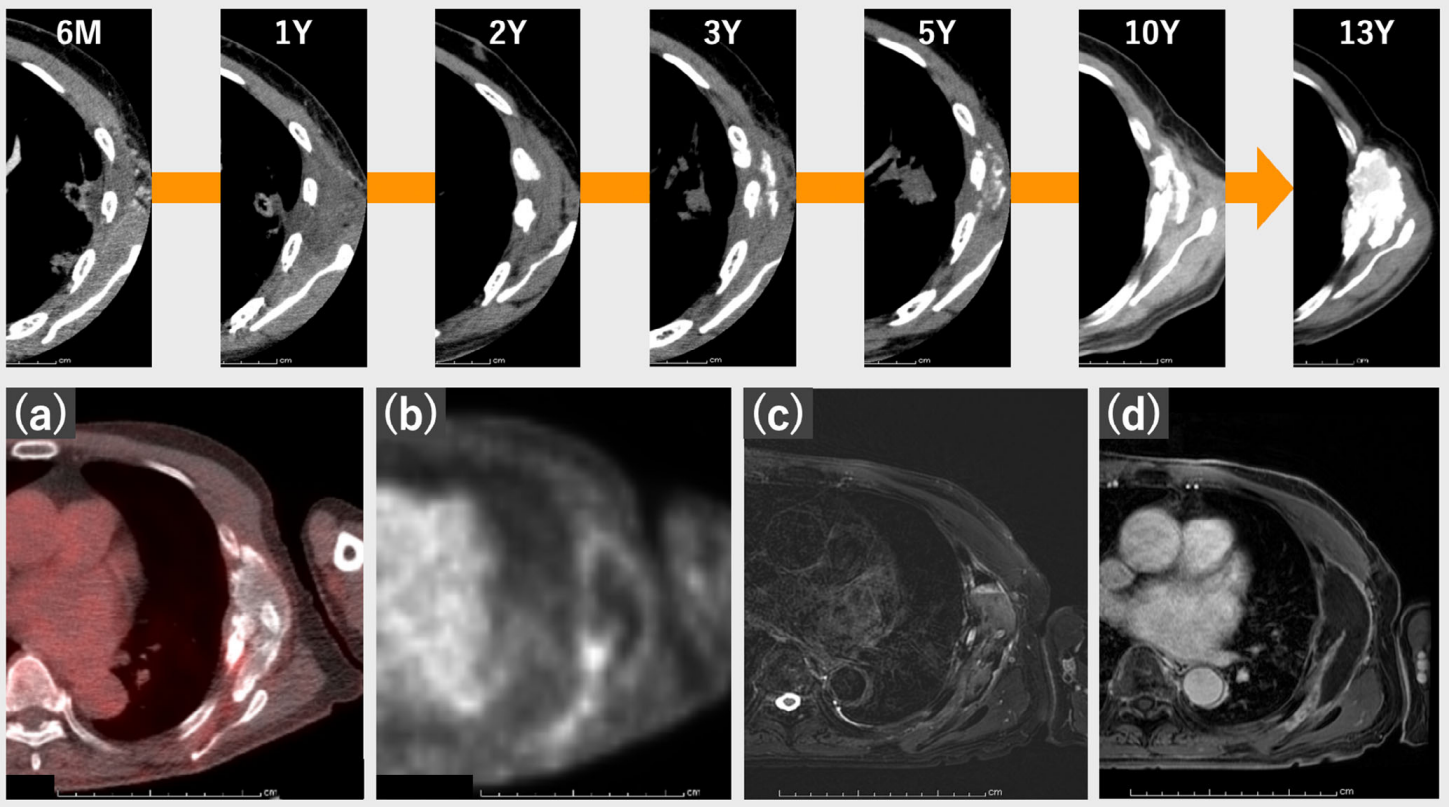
The time course of the chest wall on computed tomography (CT) imaging during the 13 years following stereotactic body radiotherapy (SBRT), showing a tumor‐like mass which increased in size and diffuse calcification. (a, b) Fluorodeoxyglucose‐positron emission tomography‐computed tomography (FDG‐PET/CT) showing low FDG uptake. (c) Fat‐saturated T2‐weighted images showing low signal intensity with fluid–fluid levels. (d) Fat‐saturated contrast‐enhanced T1‐weighted images showing no enhancement.
